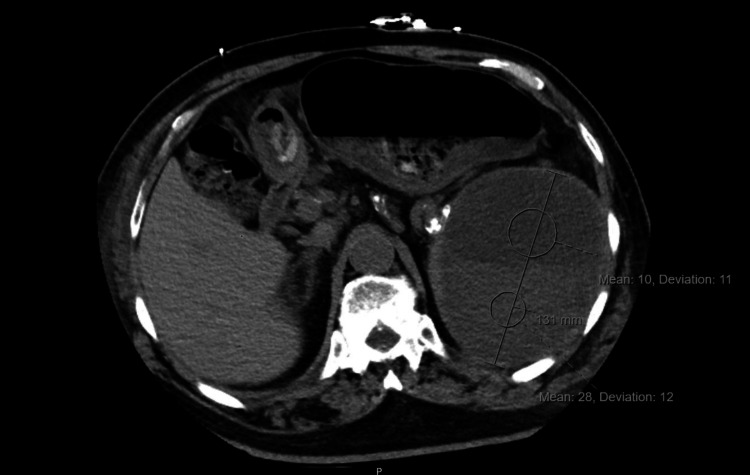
CT abdomen and pelvis reveal a new splenic fluid level
